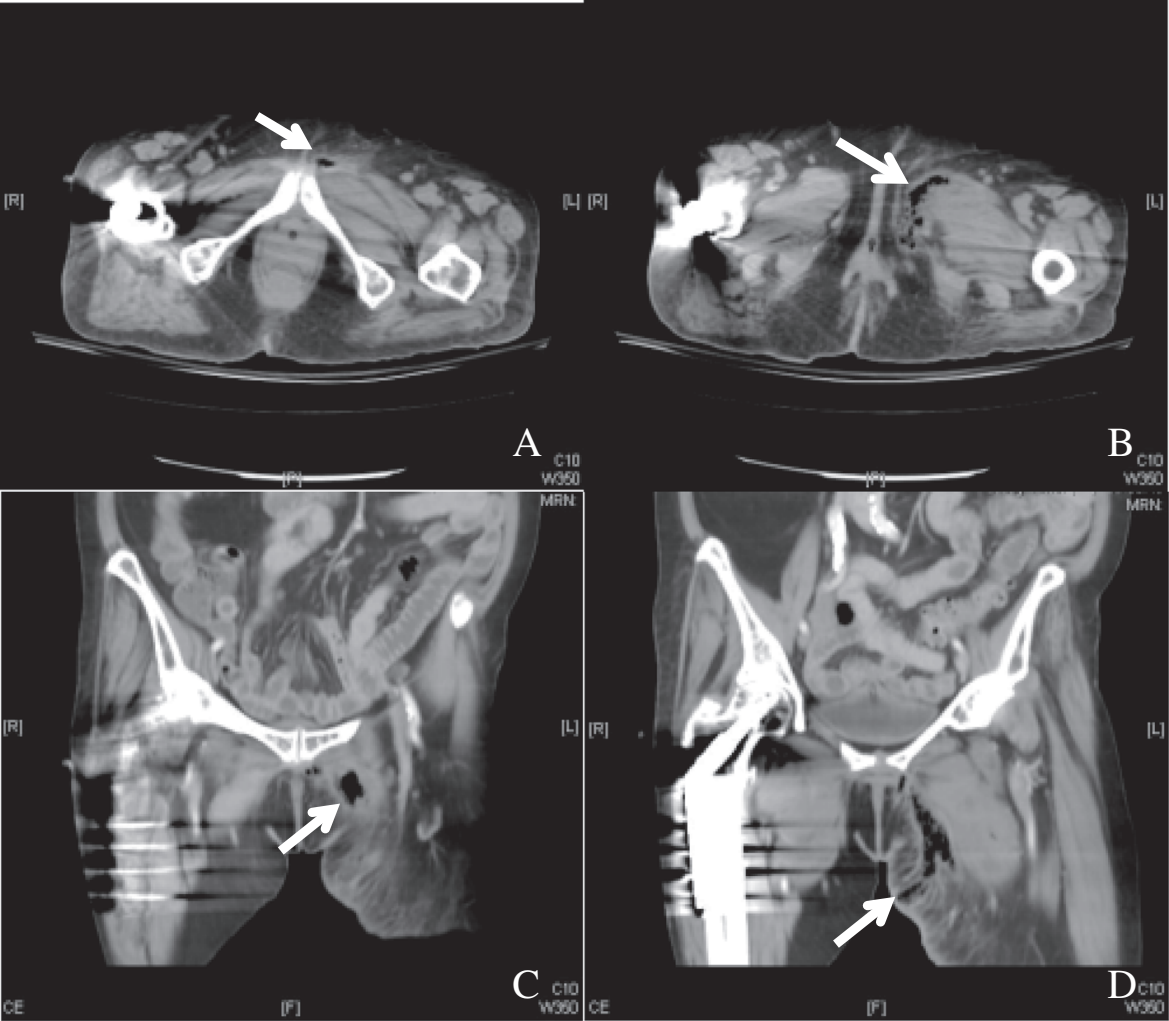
Computed tomography of the pelvic area and left thigh showed formation of an abscess with gas bubbles at groin area. Extension to the medial portion of the left thigh with blurring of the underlying adductor muscle was demonstrated. (A,B), Axial section, (C.D), Coronal section.
